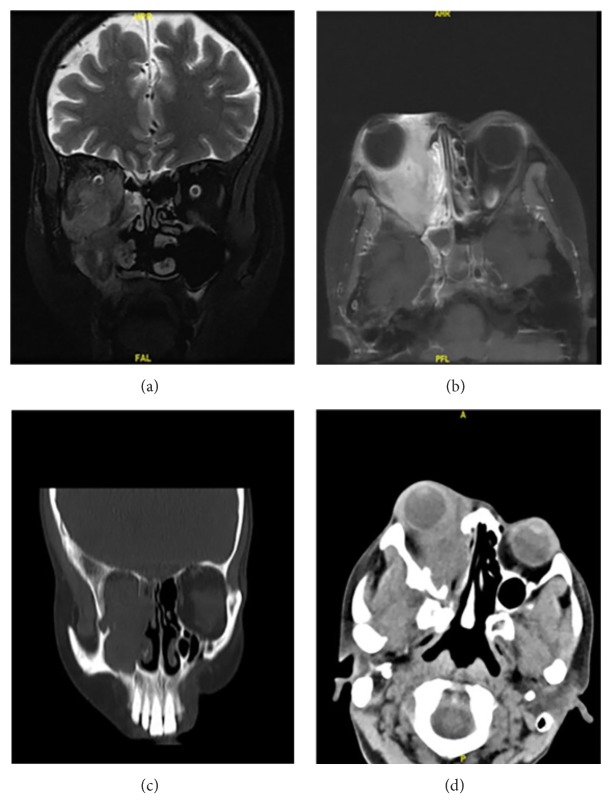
A 33-year-old woman complained of facial swelling, diplopia, and impaired vision. CT and MRI showed a soft-tissue tumor in the right maxillary sinus and orbit. Six years ago, she had been diagnosed with plasma granuloma of the right middle ear. This time she was diagnosed with an inflammatory myofibroblastic tumor and underwent endoscopy-guided incomplete resection, combined with prednisone and chemotherapy. After 1-year follow-up, her symptoms were significantly relieved. T2-weighted (a) and CT (c) coronal images showed a hyperintense and isodense mass in the maxillary sinus and orbit. Axial contrast-enhanced T1-weighted image (b) and CT (d) showed the intense enhancement of the soft-tissue tumor.
